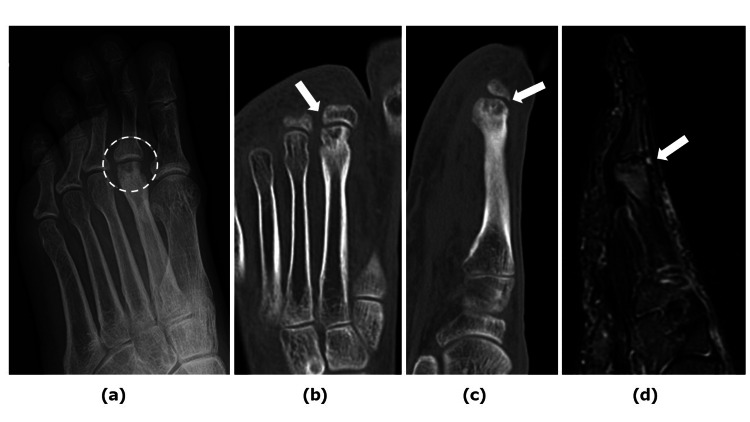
Case 2—Preoperative examination (a) Medial oblique view radiograph showing deformity and cysts of the second metatarsal head (indicated by a dotted circle) (b) Axial plane CT showing deformity and cysts of the second metatarsal head (an arrow indicates cyst) (c) Sagittal plane CT scan revealing deformity and cysts of the second metatarsal head (an arrow indicates cyst) (d) MRI T2-weighted image demonstrating high signal intensity in the third metatarsal head (an arrow indicates cyst)
